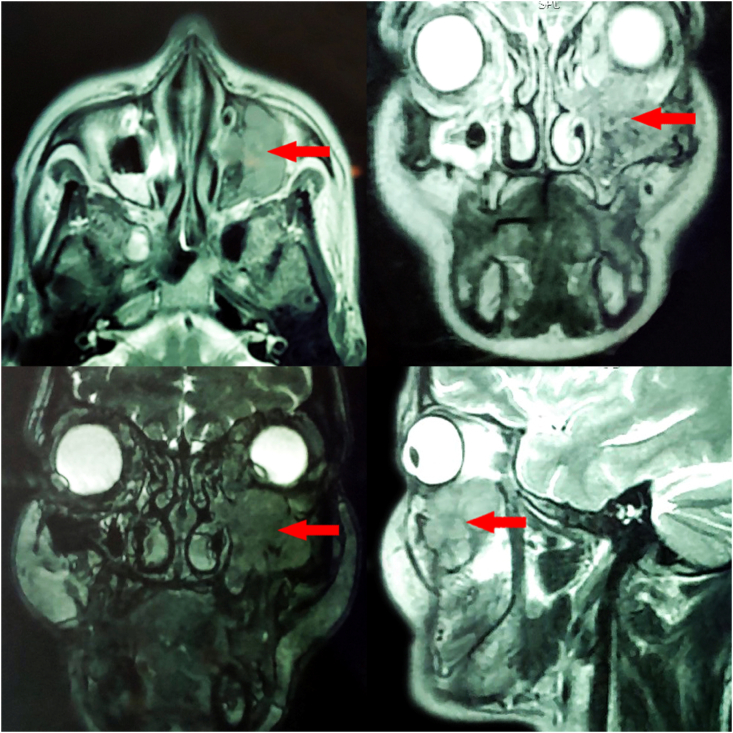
Facial MRI showing a left maxillary sinus tumor in isosignal T1 hypersignal T2 with invasion of the left orbit and nasal cavity.
